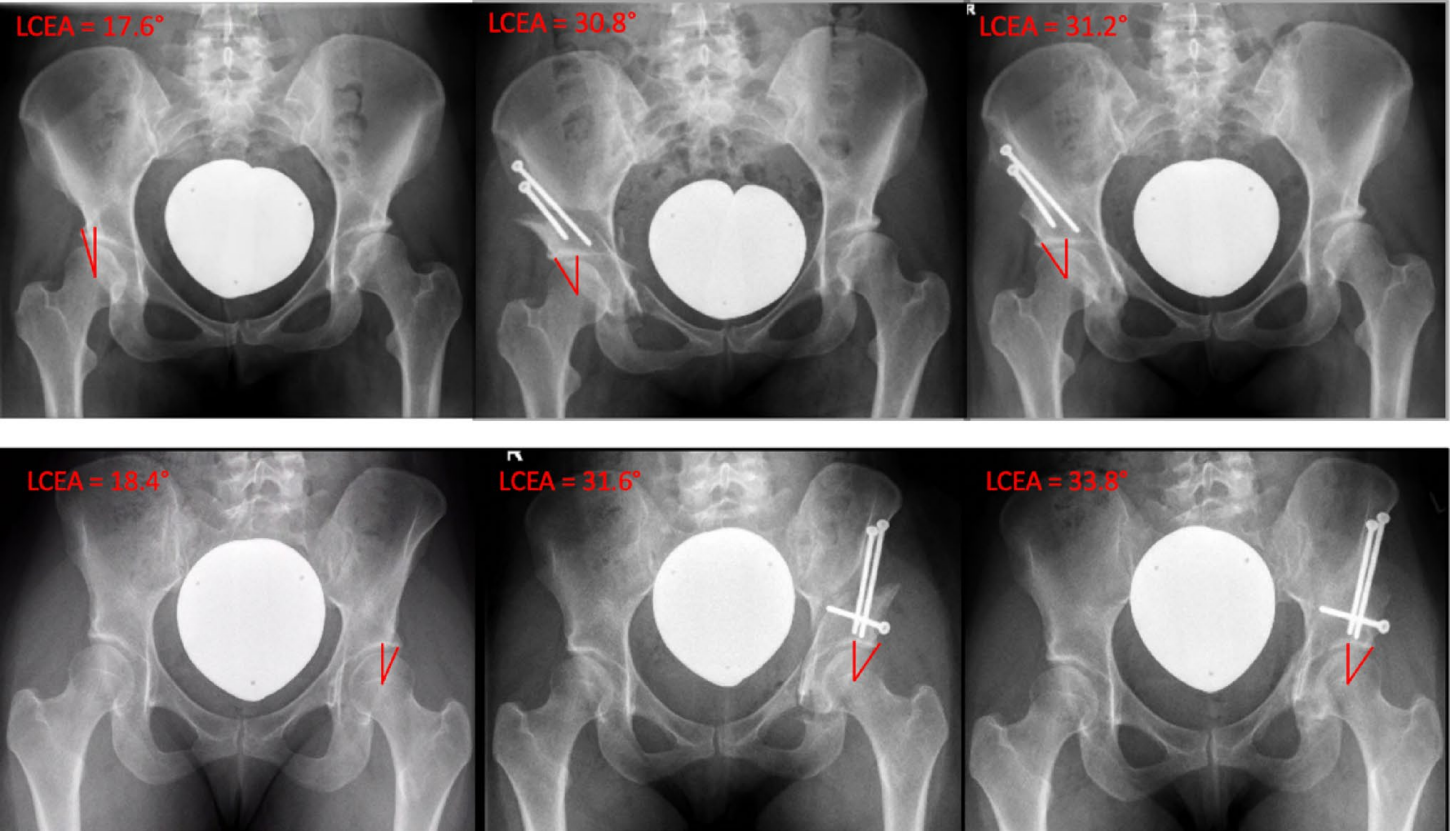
a The X-ray images show a sufficient correction of the undercoverage of the hip with two-screw fixation of the osteotomy fragment. The postoperative course demonstrates no significant change in the result of the correction one year after PAO. b In comparison, fixation of the acetabular PAO fragment with three screws also showed no significant change in the LCEA in the postoperative course up to one year postoperatively. The bony consolidation of the osteotomy gaps was also completed and comparable to the findings in (a)
